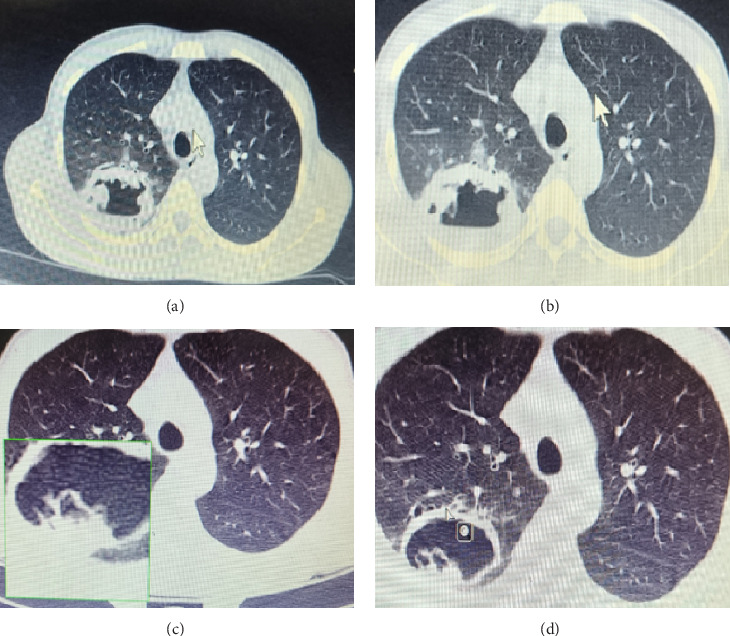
The patient's chest CT scan. (a) and (b) A thick-walled 48 ^∗^ 34 mm cavity with an air-fluid level in the posterior segment of the right upper lobe (RUL). (c) and (d) Suggested hydatid cyst.
